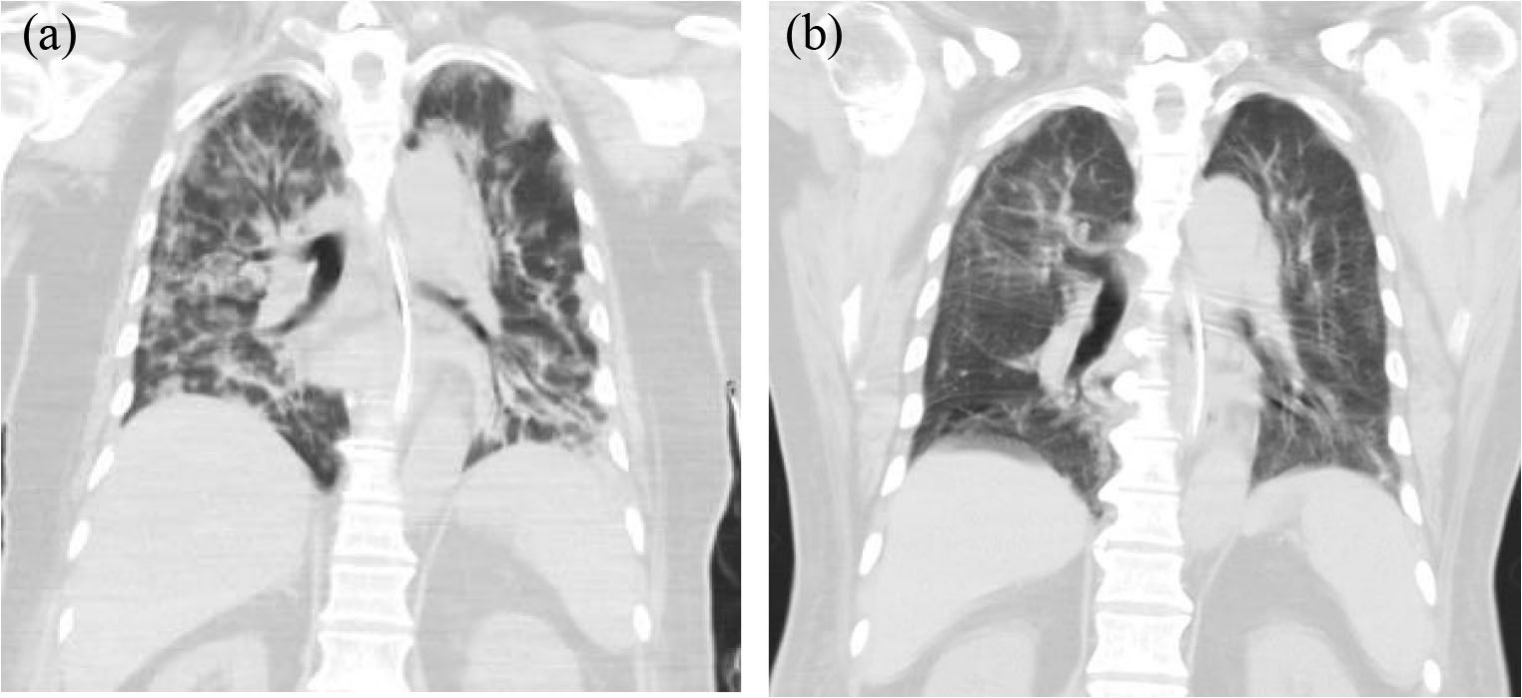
The follow-up chest CT scan of the patient. a The CT Scan on April 16 showed patchy ground glass opacity infiltrations distributed diffusely in bilateral lungs. b The CT scan on June 2 showed further regression of ground glass opacity and consolidations in the bilateral lungs
